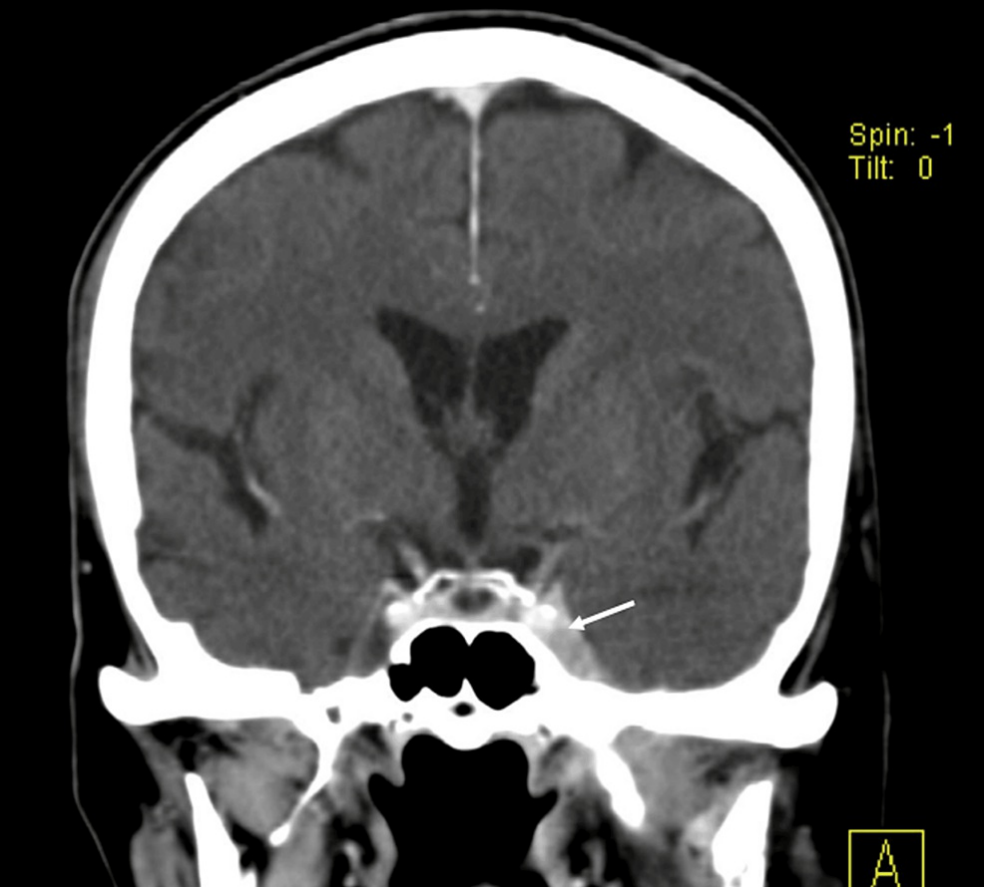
Brain computed tomography (coronal plane) showing tumor involvement of the left cavernous sinus
